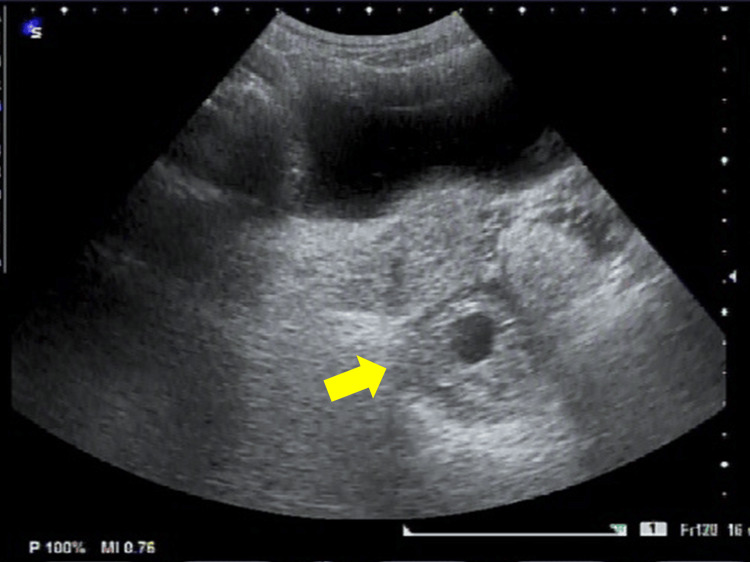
Preoperative transabdominal ultrasound. A gestational sac (arrow) is visible on the right posterior side of the uterus.
